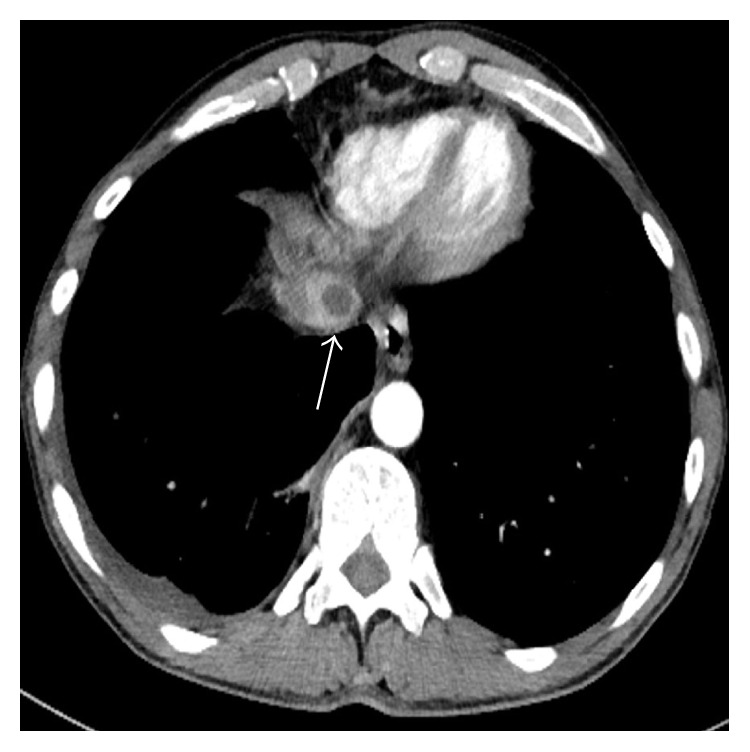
Contrast-enhanced CT scan shows a fluid attenuation round lesion, daughter cyst, within the IVC (arrow).
